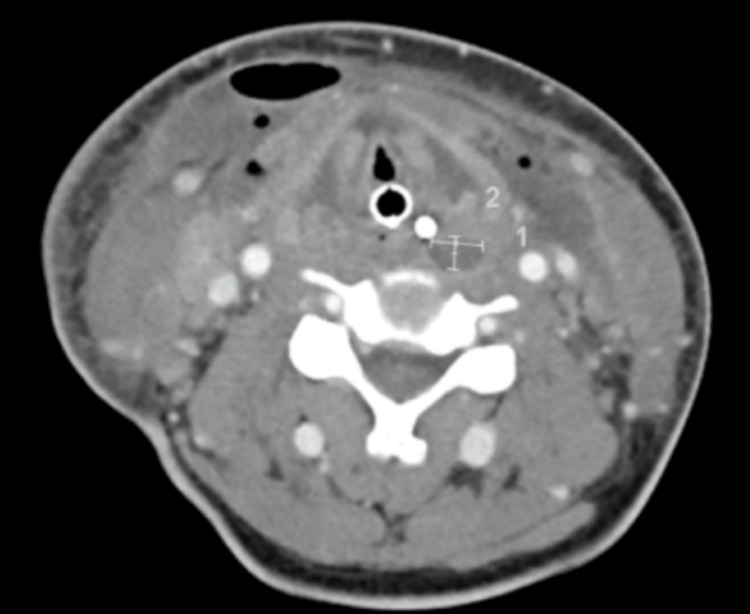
Repeat CT scan obtained on day five showing the neck abscess
